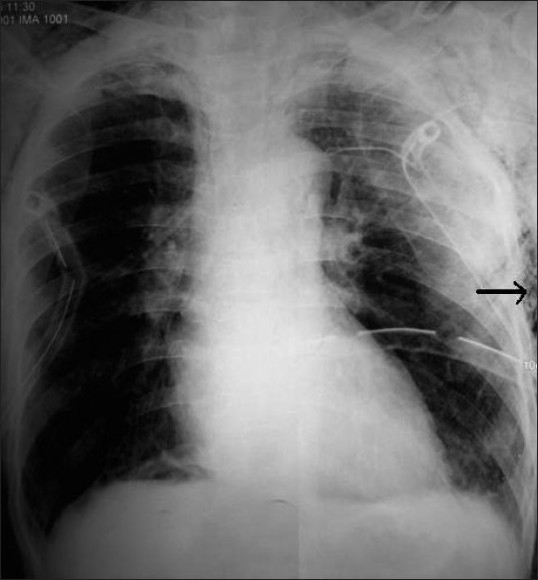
X-ray chest with bilateral intercostal drains; the arrows depicting areas of subcutaneous emphysema
